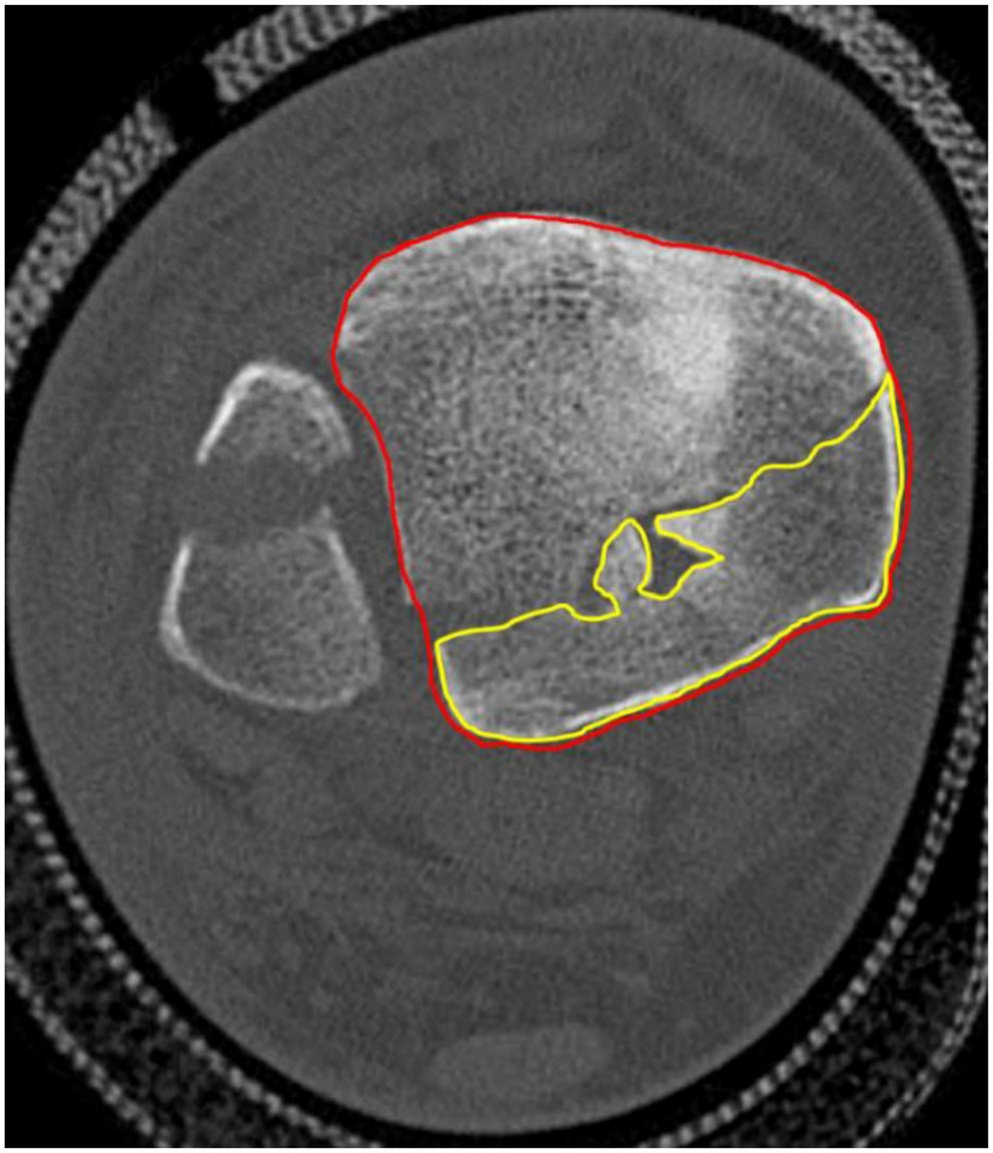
In the method 2 the PMF area is divided by the total articular surface of the tibia on the axial view of the CT then expressed in %
